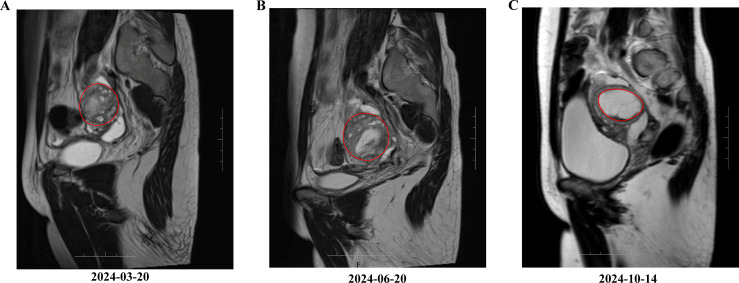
Pelvic MRI of the left ovarian mass from March to October 2024. (A) March 2024: Sagittal T2-weighted fast spin-echo sequence (TE 83 ms, TR 5390 ms, slice thickness 4.0 mm) showing a 5.7×4.5×3.8 cm multicystic lesion in the left adnexal region (red circle) with internal septations and features suggestive of hemorrhage. (B) June 2024: Sagittal T2-weighted fast spin-echo sequence (TE 83 ms, TR 4600 ms, slice thickness 4.5 mm) showing a 3.7×3.7×4.7 cm multicystic lesion in the left adnexal region (red circle) with internal septations and signal characteristics suggestive of hemorrhage. (C) October 2024: Sagittal breath-hold T2-weighted turbo spin-echo sequence (TE 80 ms, TR 850 ms, slice thickness 4.5 mm) showing a 5.7×4.5×3.8cm cystic lesion in the left adnexal region (red circle), suggestive of hemorrhage.
